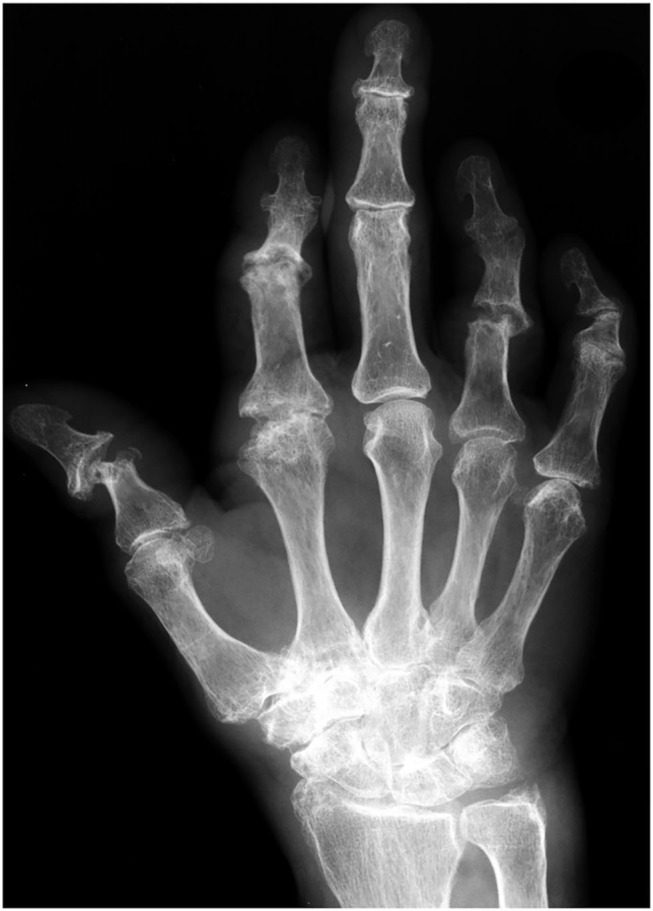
Plain x-ray of the right hand with arthritis mutilans.
